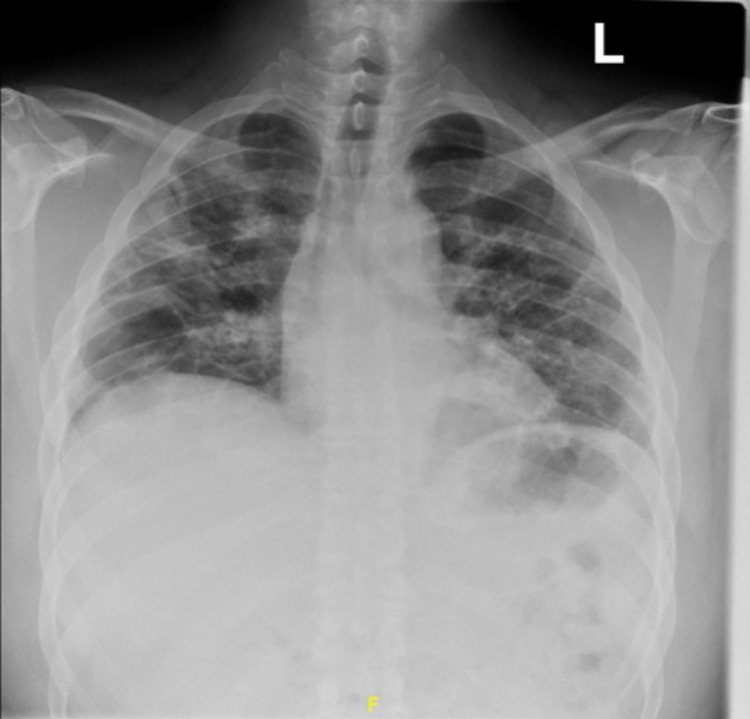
A chest X-ray was taken one month after hospitalization without clinical or radiographic signs of pneumothorax.
